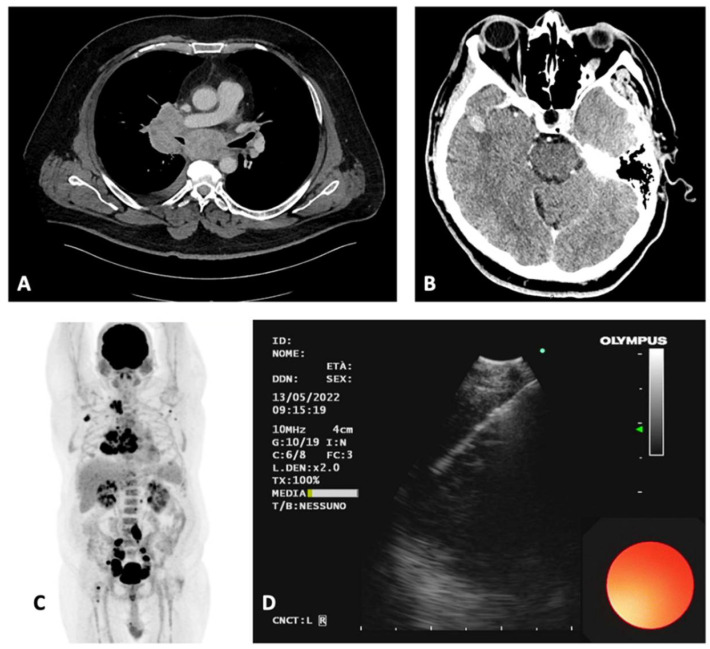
Case 1. (A) Axial contrast-enhanced CT scan showing multiple mediastinal adenopathies. (B) encephalic CT showing a right temporal lobe metastasis. (C) FDG-PET scan showing uptake of the mediastinal and abdominal lymphoadenopathies. (D), EBUS-TBNA of the subcarinal lymph node, station 7 according to IASLC. CT, computerized tomography; FDG- PET, fluorodeoxyglucose positron emission tomography; EBUS-TBNA, endobronchial ultrasound with transbronchial needle aspiration; IASLC, International Association for the Study of Lung Cancer.
